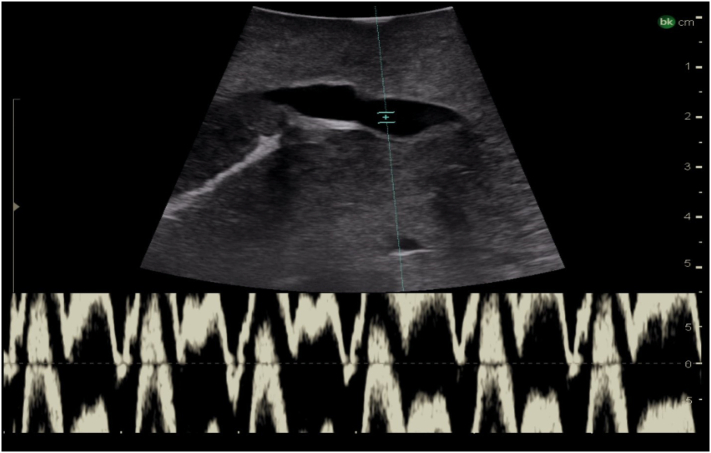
Intraoperative Doppler ultrasound of hepatic venous flow.
